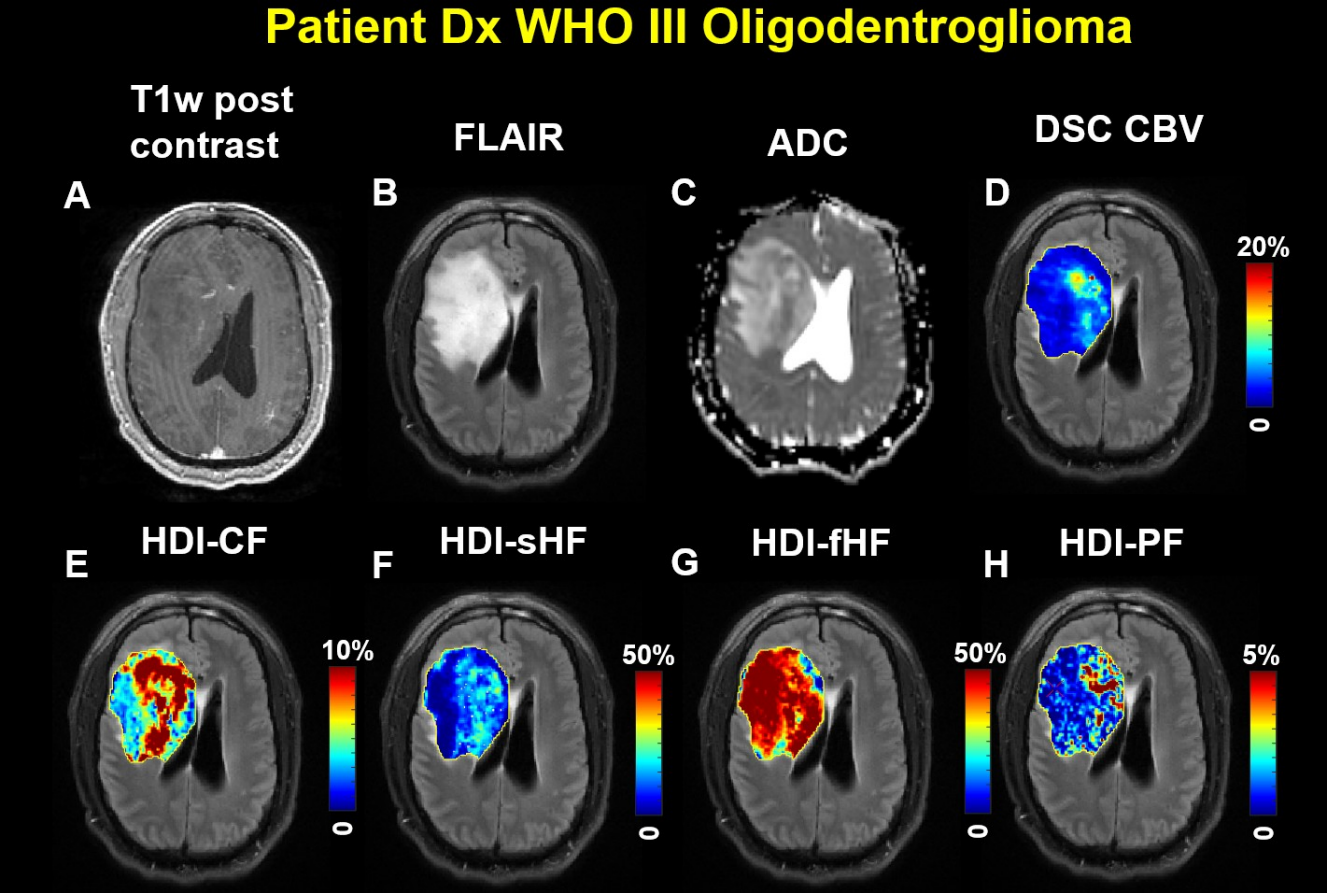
Imaging from a man in his 50s diagnosed with World Health Organization grade III oligodendroglioma. (A) The T1-weighted post-contrast image shows a lesion with decreased signal intensity. (B) The fluid-attenuated inversion recovery image and (C) the diffusion magnetic resonance imaging-derived apparent diffusion coefficient show a lesion with an increased signal. (D) The dynamic susceptibility contrast-derived cerebral blood volume map and the Heterogeneity Diffusion Imaging-derived (E) cellularity fraction, (F) slow hindered diffusion fraction, (G) fast hindered diffusion fraction, and (H) perfusion fraction maps were generated on manually defined tumor regions of interest and overlaid on the fluid-attenuated inversion recovery image. The elevated cerebral blood volume and Heterogeneity Diffusion Imaging-derived cellularity fraction, slow hindered diffusion fraction, fast hindered diffusion fraction, and perfusion fraction are shown in the tumor region.
